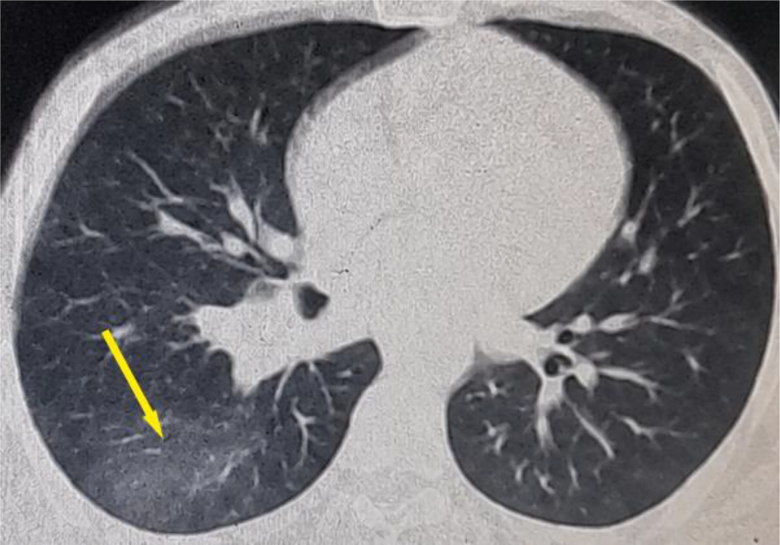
Chest CT scans (lung window). The images display parenchymal abnormalities, including consolidation in the right lung fields. CT = computed tomography.
